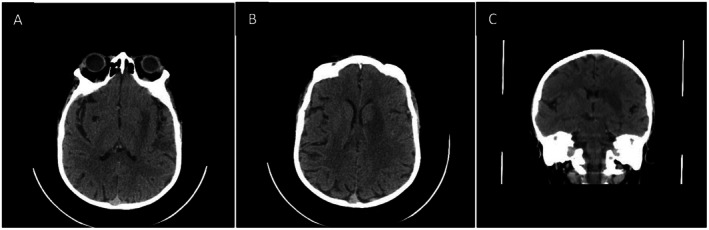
Non‐contrast brain CT (A–C). Axial non‐contrast CT images showing no acute hemorrhage. Subtle hypodensity is noted in the left basal ganglia and insular region (arrows), raising suspicion for early ischemic change.
